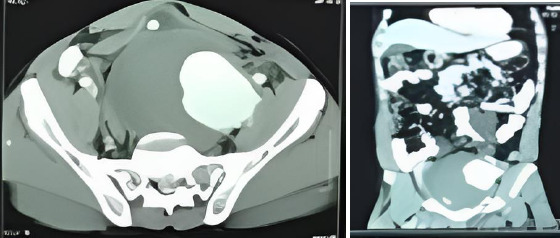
Pre-operative CT angiogram of Case 1
